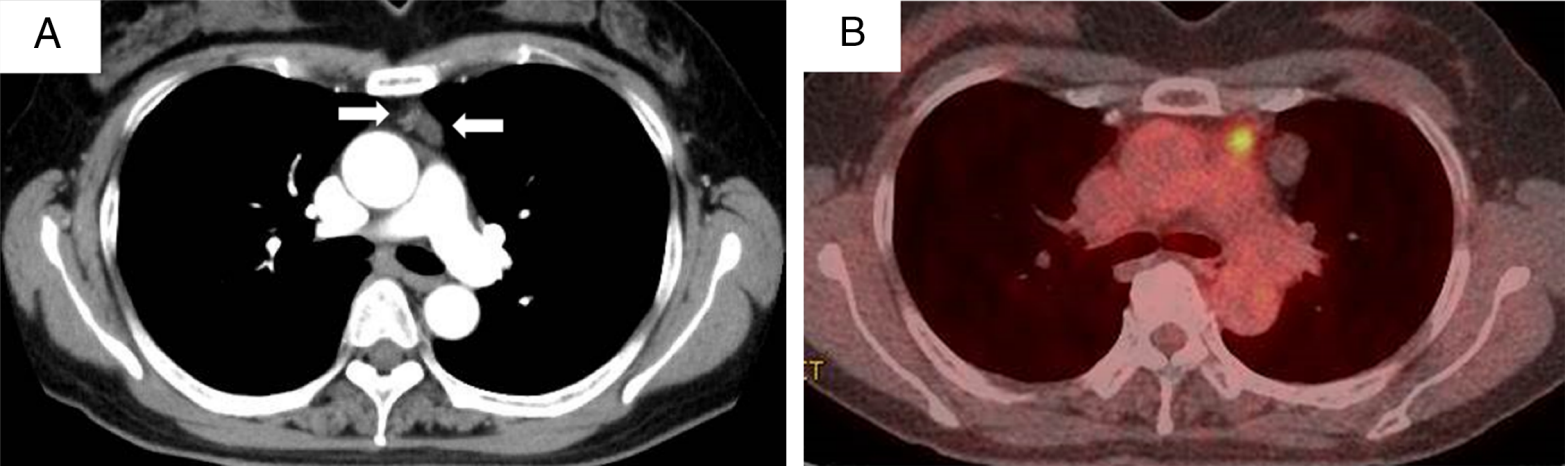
(A) Chest computed tomography (CT) showing a 35‐mm, well‐circumscribed cyst (arrow) and an adjacent 10‐mm nodule (arrow) in the anterior mediastinum. (B) FDG‐PET/CT showing increased uptake only in the 10‐mm nodule (arrow) in the anterior mediastinum (SUVmax 3.8).
